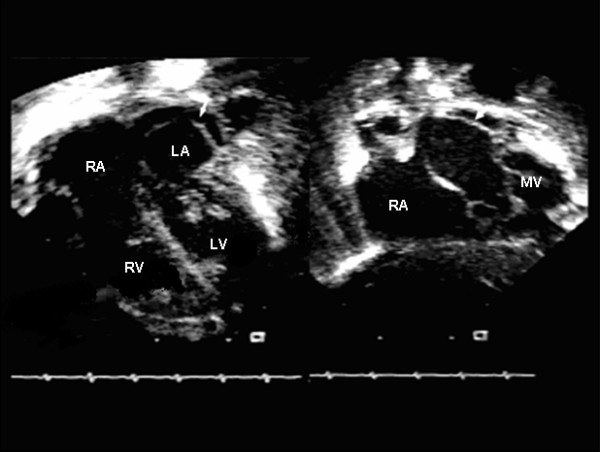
Apical four chamber and subcostal views. Note the membrane (arrow) extending from the level of the mitral valve to the upper pulmonary veins. RA; right atrium, LA; left atrium, RV; right ventricle, LV; left ventricle, MV; mitral valve.
